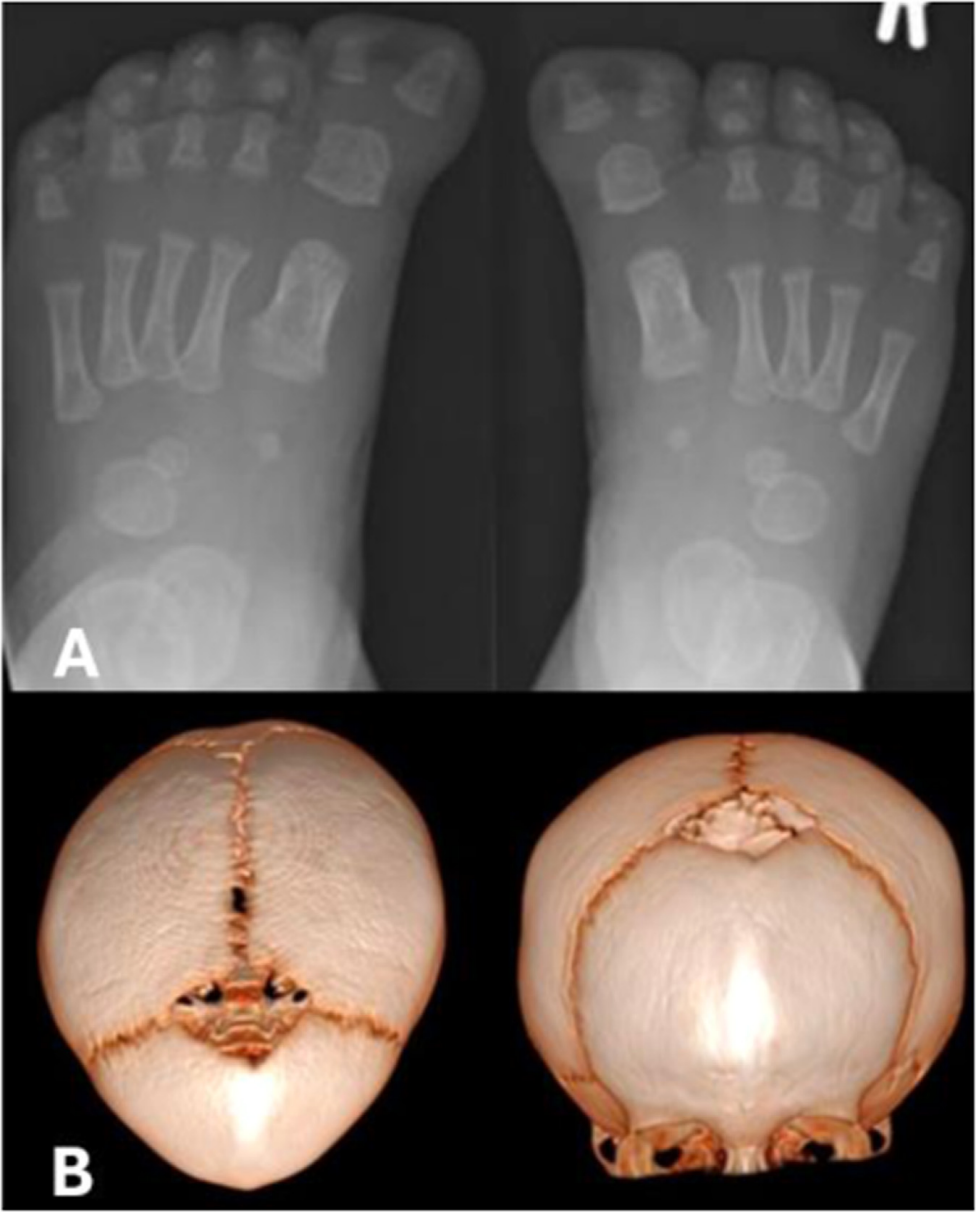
(A) Left and right foot x-rays showing a duplicated distal phalanx of each great toe. (B) 3D reconstruction of computed tomography scan. Trigonocephalic appearance with triangular forehead, absent/fused metopic suture and metopic ridge.
